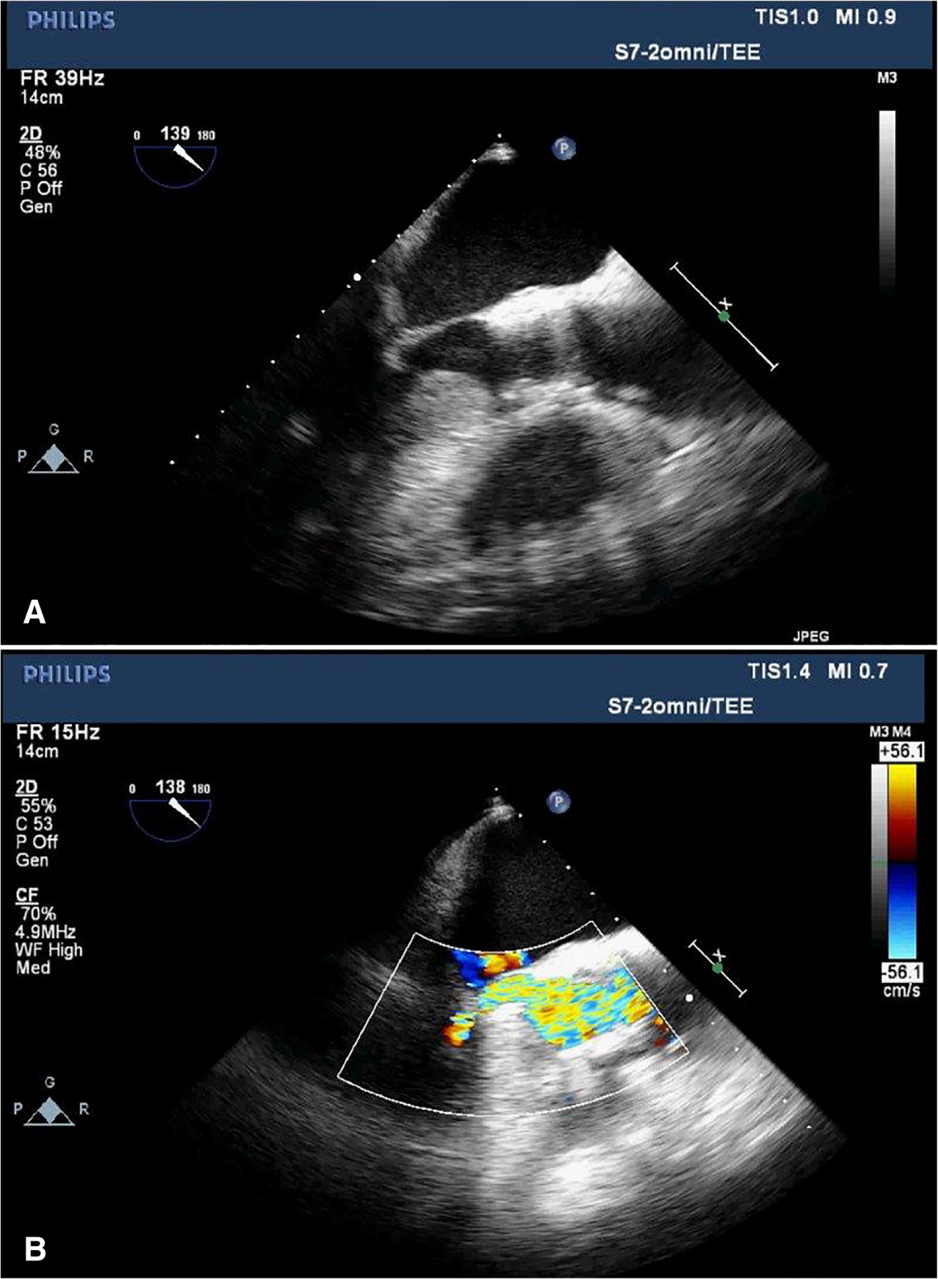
Intraoperative transesophageal echocardiogram, mid-esophageal long-axis view obtained after rapid pacing and valve deployment demonstrating (a) systolic anterior motion of the mitral valve leading to severe restriction of flow (b) as demonstrated on color Doppler imaging
